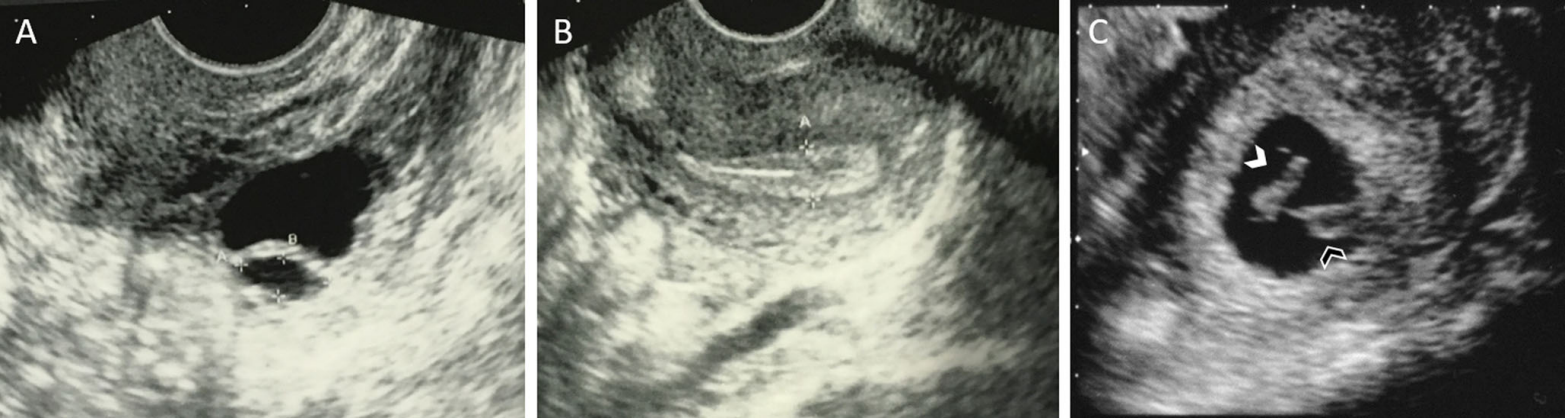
Transvaginal ultrasound scan images post-re-implantation. a Two antral follicles on the left ovary seen at 29 weeks postoperatively. The larger follicle had a mean diameter of 15 mm and ovulation was confirmed by a progesterone rise 1 week later. b Longitudinal view of the uterus at 29 weeks post-re-implantation demonstrating an endometrial thickness of 7.9 mm. c Single viable intrauterine pregnancy at 7^+3^ weeks gestation by crown-rump length (CRL) measurement (*white arrow*). The yolk sac is denoted by the *black arrow*. A corpus luteum was seen on the left ovary
